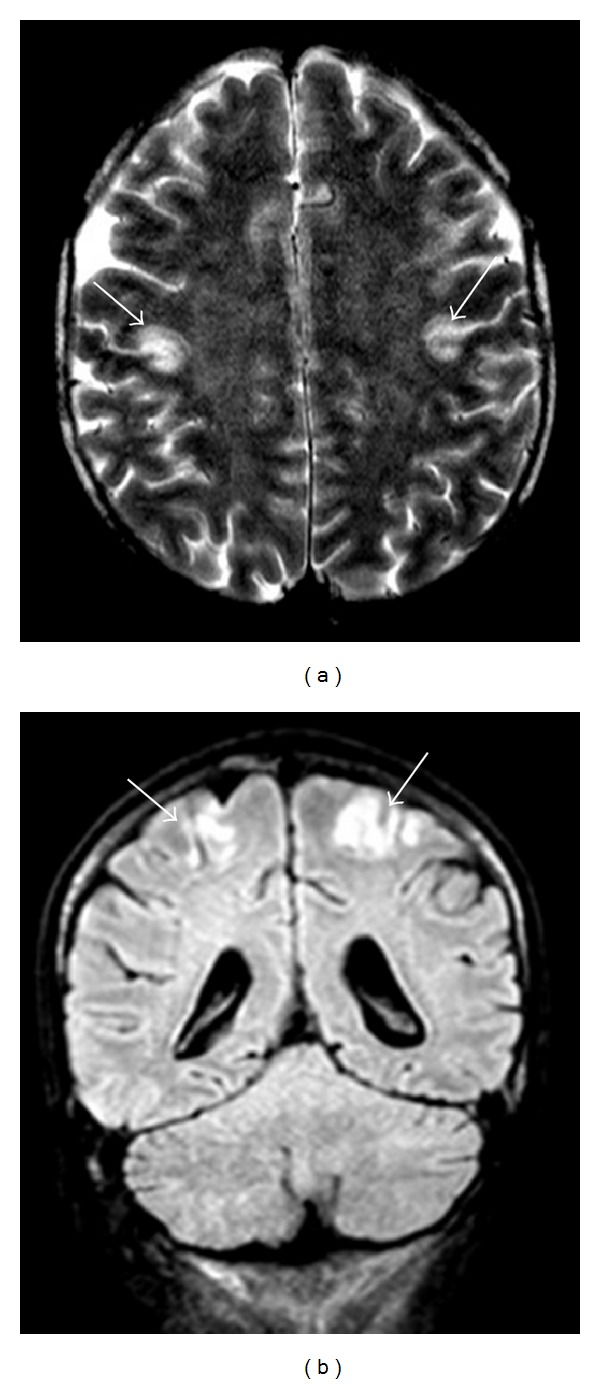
Forty-five-year-old man, affected by acute lymphoblastic leukemia, under chemical therapy, experiencing nausea and oral pain with consequent anorexia. T2-weighted axial (a) and FLAIR coronal (b) images showing bilateral hyperintense signal alteration at the level of the fronto-parietal cortex.
